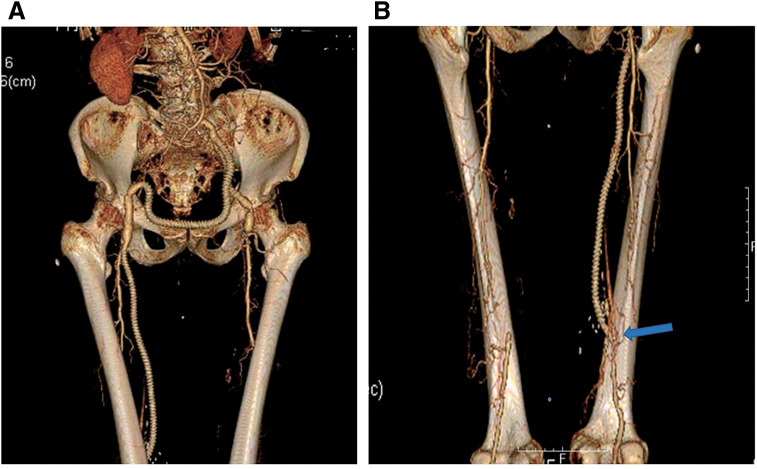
Fig. 3 An emergency case of above knee F-P bypass and multiple inflow procedures for the treatment of acute limb ischemia (Patient 5). (A) 3D reconstruction of postoperatively obtained CT showing a patent PTFE graft for a left EIA-CFA bypass and left CFA-right CFA bypass. (B) 3D reconstructed posterior view of a PTFE graft above the right knee for an F-P bypass. The blue arrow indicates the site of the vein cuff. 3D: three-dimensional; CFA: common femoral artery; CT: computed tomography; EIA: external iliac artery; F-P: femoropopliteal; PTFE: polytetrafluoroethylene
